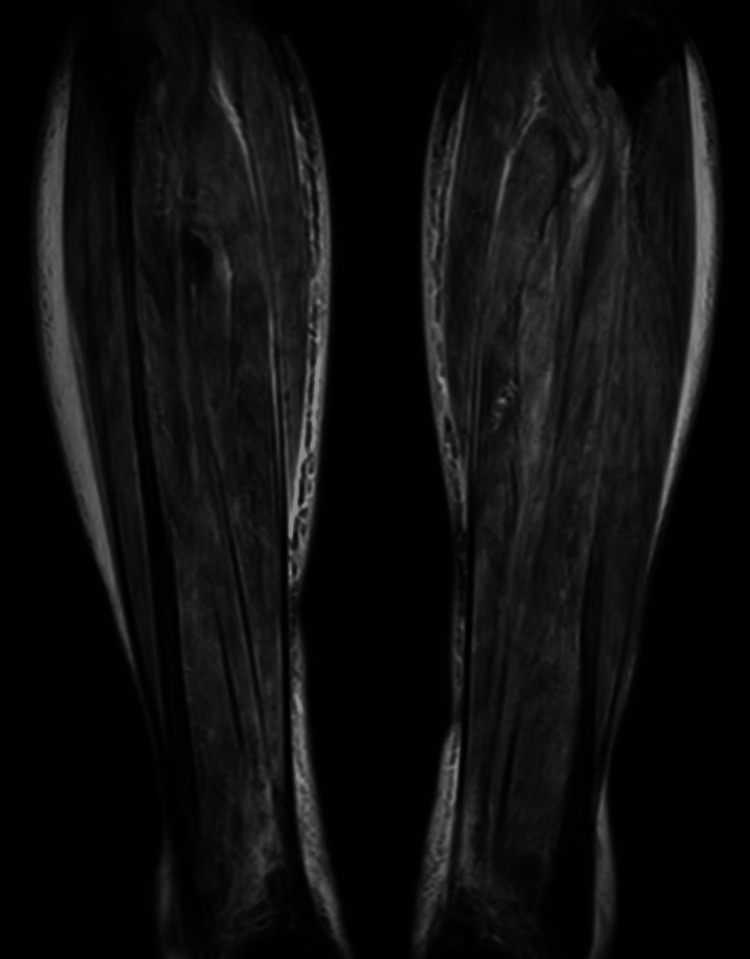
Magnetic resonance imaging of the bilateral triceps surae muscles. Magnetic resonance imaging of the leg revealed high signal intensity in the bilateral triceps surae muscles on T2 fat-saturated images.
